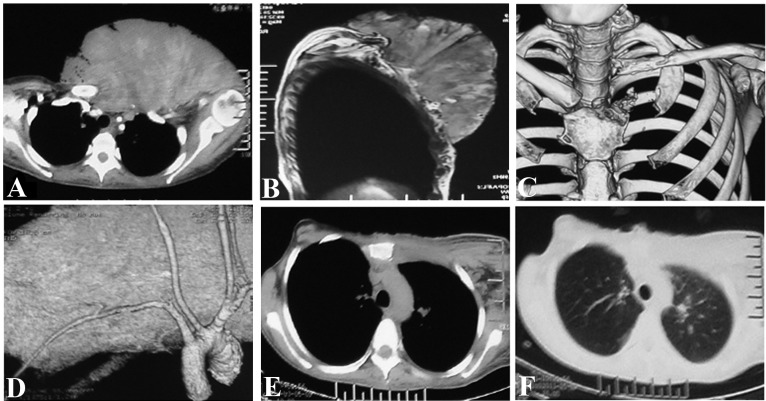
(A) Computed tomography (CT) and (B) magnetic resonance imaging showing an expansive mass in the left anterior-superior chest wall with an irregular margin, but with no evidence of invasion of the pleural cavity. (C) Three-dimensional imaging of the thoracic cage showing tumor invasion of the left clavicle, the first rib, superior segment of the sternum and the sternoclavicular joint. (D) The CT angiography indicated that the main blood supply of the tumor came from the proximal end of the left subclavian artery, particularly the internal thoracic artery. (E and F) The thoracic CT was rechecked and no signs of a remaining tumor were detected.
